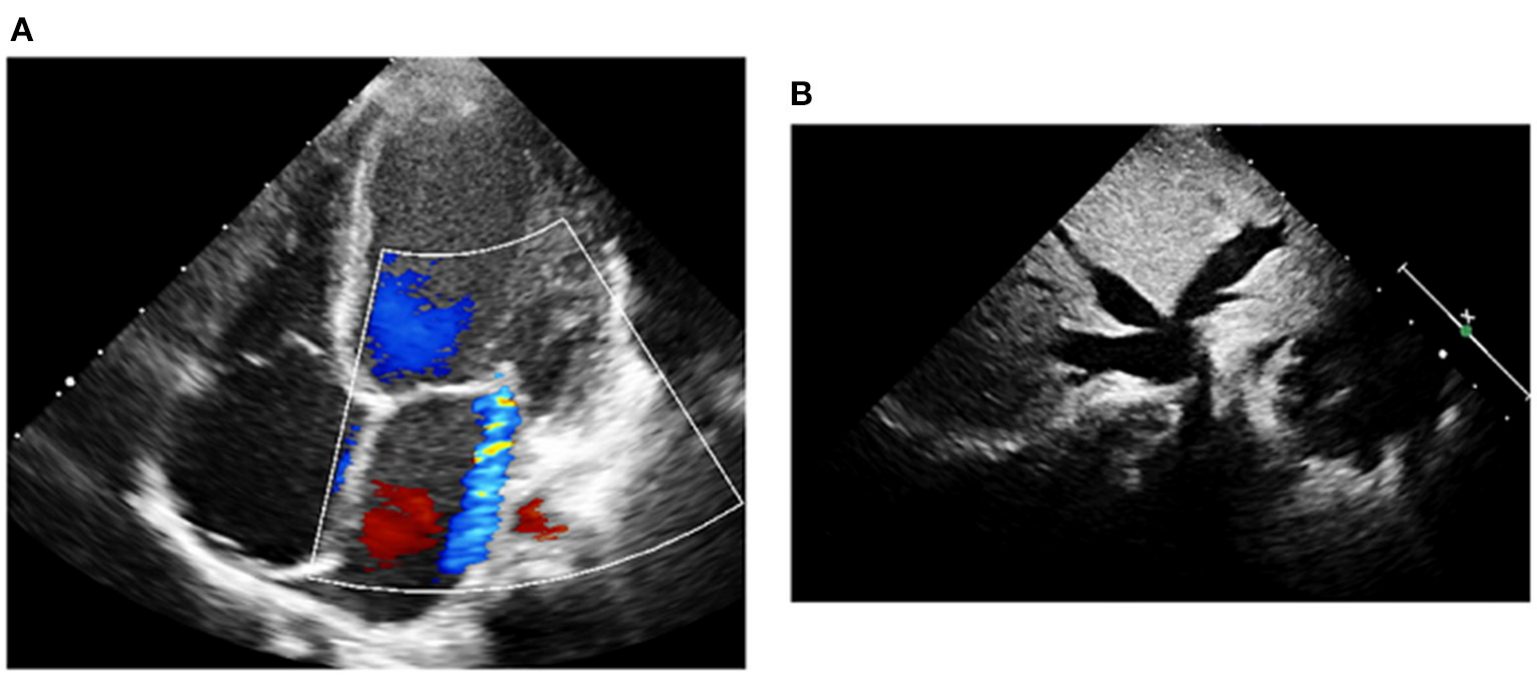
Echocardiography of patient #1 prior to treatment with ivabradine. (A) A moderate mitral regurgitation and a severely dilated left ventricle were observed on echocardiography. (B) Liver ultrasound revealed dilated inferior vena cava and hepatic veins.
